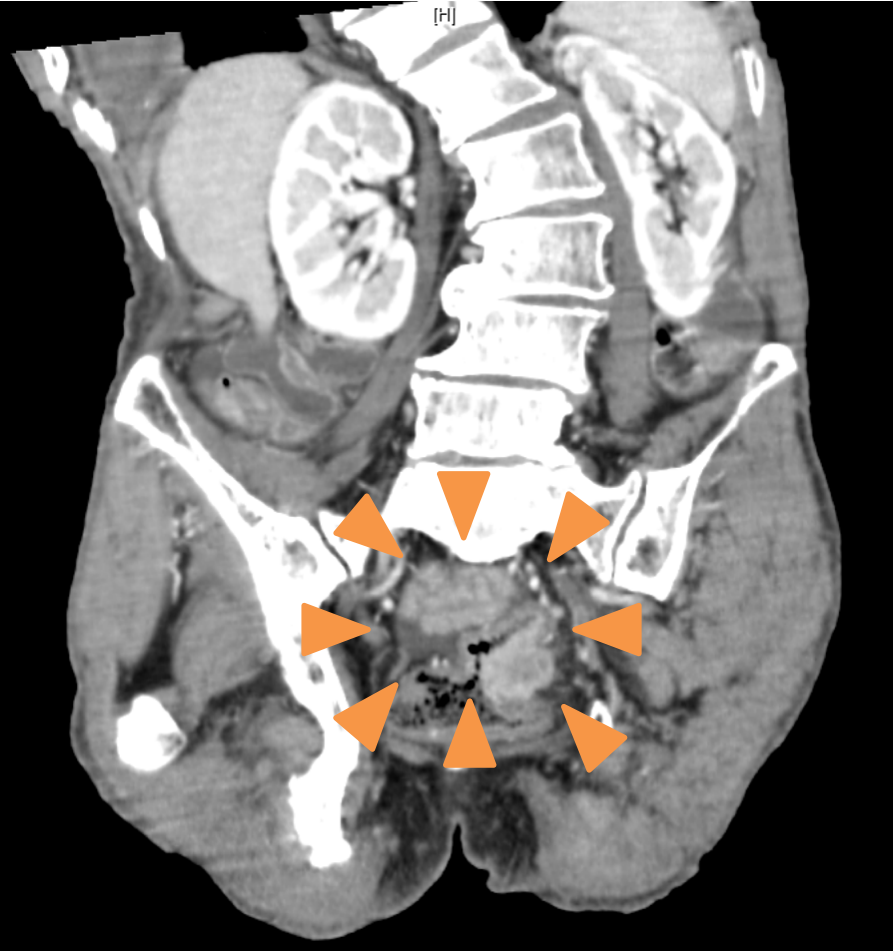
An abdominal computed tomographic scan revealing a mass lesion in the rectum (*orange arrowheads*)
